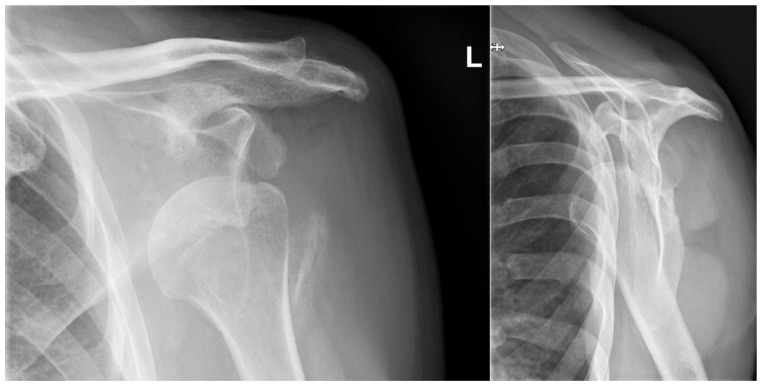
Preoperative radiographs. Anteroposterior and lateral “Y-view” radiographs showing the anterior glenohumeral dislocation of the affected shoulder.
